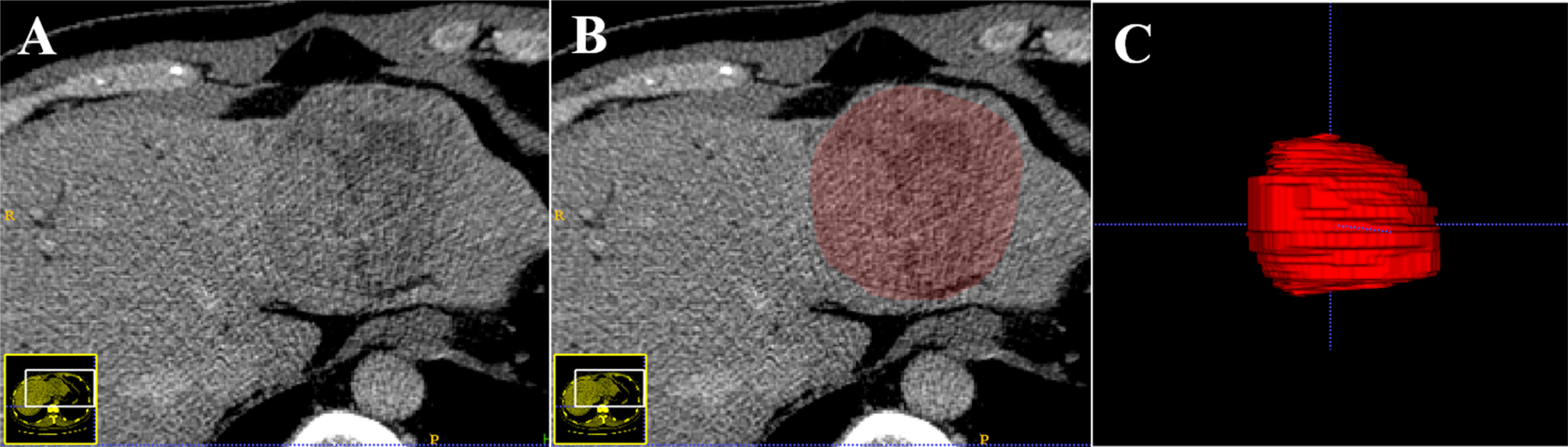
An example of the manual segmentation in hepatocellular carcinoma. The portal venous phase computed tomography (CT) image (A). Manual segmentation on the same axial slice (B). Generation of a 3D ROI (C).
